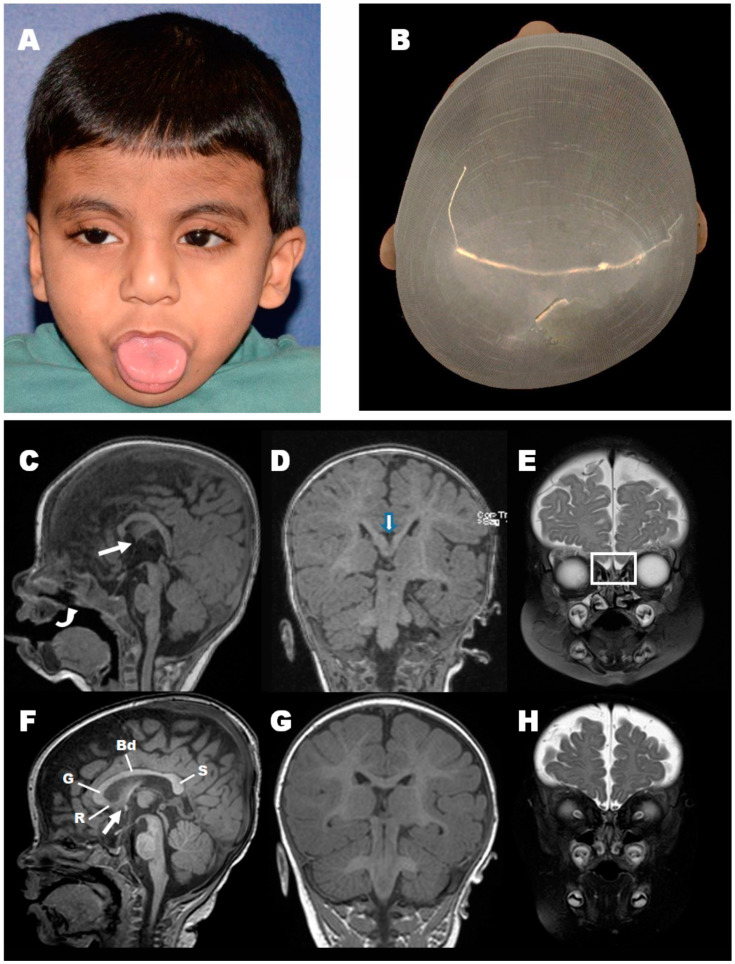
Reported case clinical presentation and Magnetic Resonance Imaging findings. Facial photos of the presented patient at 45 months of age. (A) Note the congenital blepharoptosis, tongue thrust posture, mild left ear constriction, mild frontal hirsutism. (B) Plagiocephalic head shape. (Note: The key clinical finding of cleft palate is not visible in these images). (C) Brain MRI showing midline sagittal T1 weighted image demonstrates a dysplastic corpus callosum with an atypical configuration showing focal thinning at the genu of the corpus callosum and an elongated vertically oriented splenium of the corpus callosum. The anterior commissure is hypoplastic (thin arrow). Cleft Palate is also noted (curved arrow). (D) Coronal T1 weighted image shows a cleft at the level of the splenium (thick arrow) denoting partial agenesis. (E) Coronal T2 weighted image shows the absence of the olfactory bulbs (Rectangle). (F–H) Similar views of an age match control show the normal configuration of the corpus callosum, which is divided into four parts: Rostum (R), genu (G), Body (Bd), and Splenium (S) as well as the anterior commissure (thin arrow). Note the olfactory bulbs on the coronal T2 weighted image (F).
